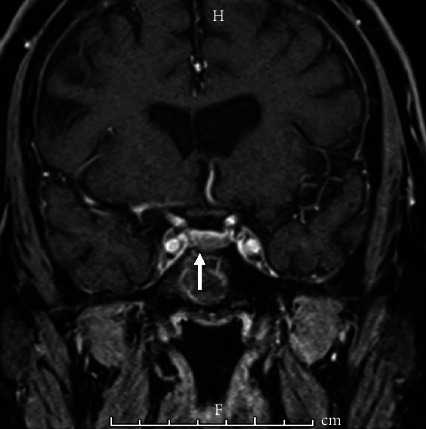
MRI of pituitary microadenoma consistent with Cushing's disease. Coronal T1-weighted enhanced MRI of the pituitary gland shows an anterior inferior pituitary lesion to the right of midline measuring approximately 6 × 6 × 3 mm.
